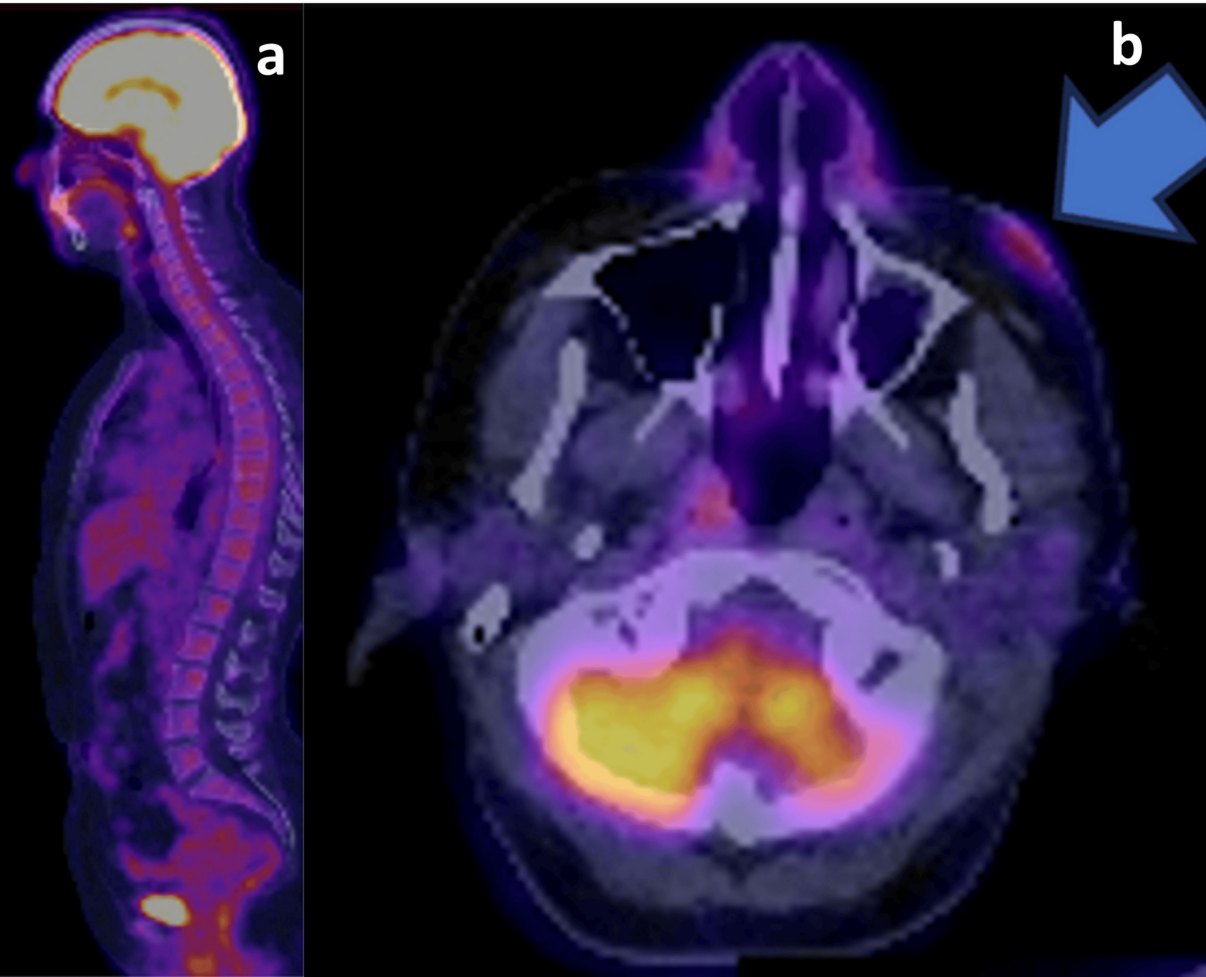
a) Sagittal view of FDG-PET/CT. b) Avidity of the soft tissue of the malar region (blue arrow). FDG-PET/CT: fluorodeoxyglucose positron emission tomography/computed tomography
